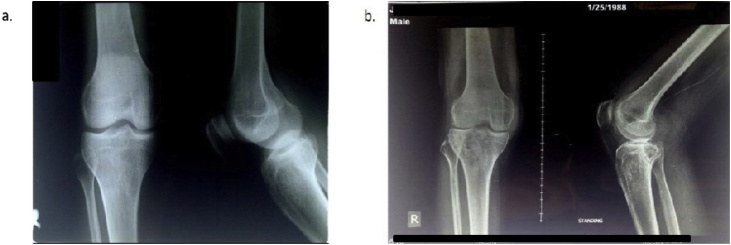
Knee Radiograph. (a) In 19 November 2013, it is shown the narrowing of knee joint gap. (b) In 18 January 2016, it can be observed that there has been a lytic lesion with ill-defined in periarticular projection in right proximal tibia and there was an ill-defined focal thickening in suprapatella and posterior of the right knee suspected a primer bone lesion in peri-articular proximal tibia
